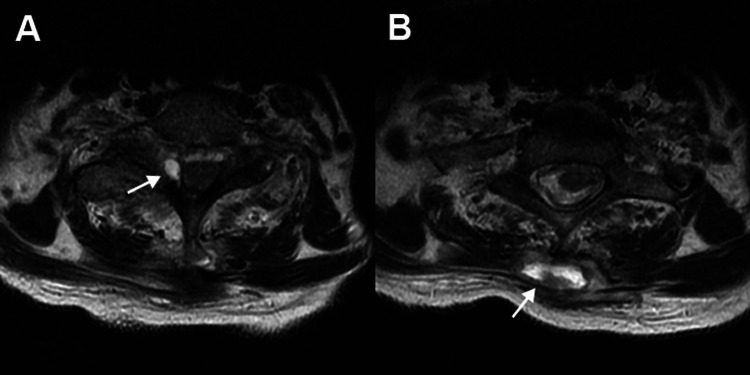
Axial MRI scan of patient's cervicothoracic spine. Axial MRI showing: (A) cervical-thoracic epidural phlegmon and (B) cervical-thoracic paraspinous process phlegmon.
